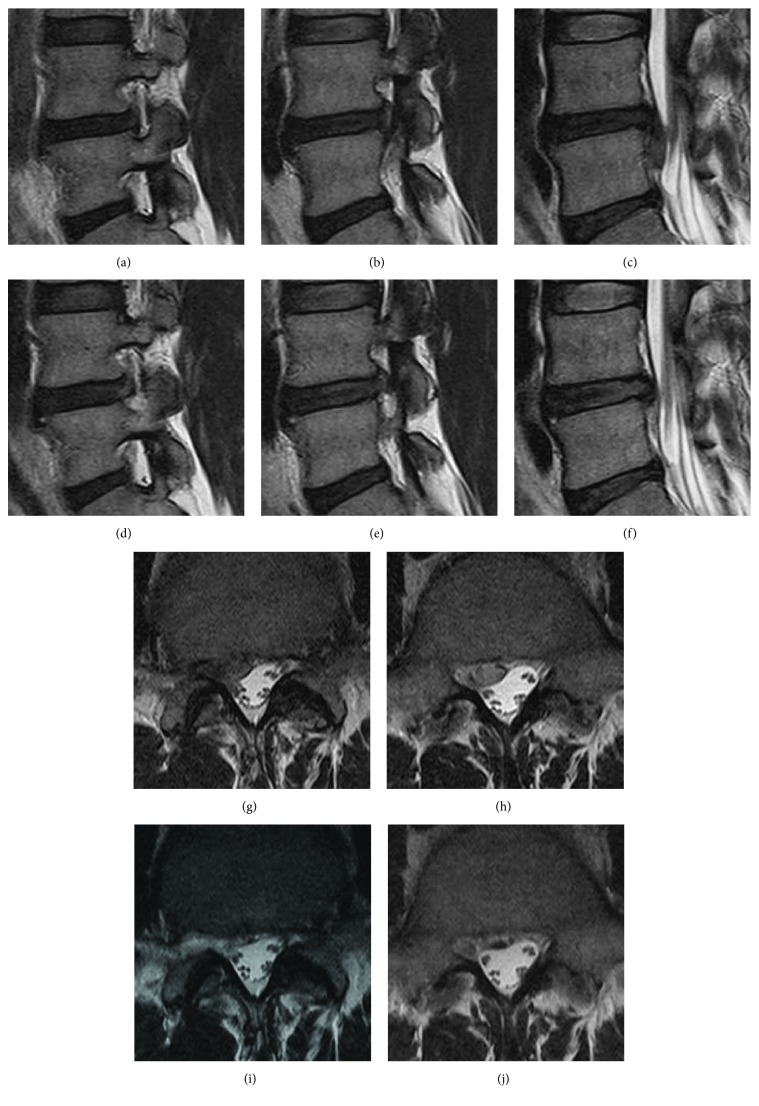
Representative case serial MRI of SCOT. (a), (b), (c), (g), (h) Preoperative MRI showing high grade inferior migration at L4-5 level. (d), (e), (f), (i), (j) Postoperative MRI showing complete removal of high grade inferiorly migrated herniated disc. (a), (d) Entry part of SCOT, (b), (e) middle part of SCOT, and (g), (h) final part of SCOT.
